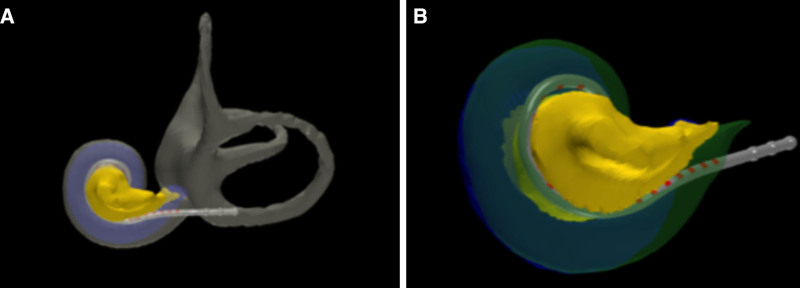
Computed tomography (CT) scan analysis of electrode array placement following cochlear implantation. *A*, CT scan analysis with a perimodiolar electrode array including the cochlear, vestibule, and semicircular canals. *B*, Closer visualization of the electrode array and cochlea allows for assessment of the electrode array position with the modiolus (yellow) and scala tympani (blue) and scala vestibuli (green).
